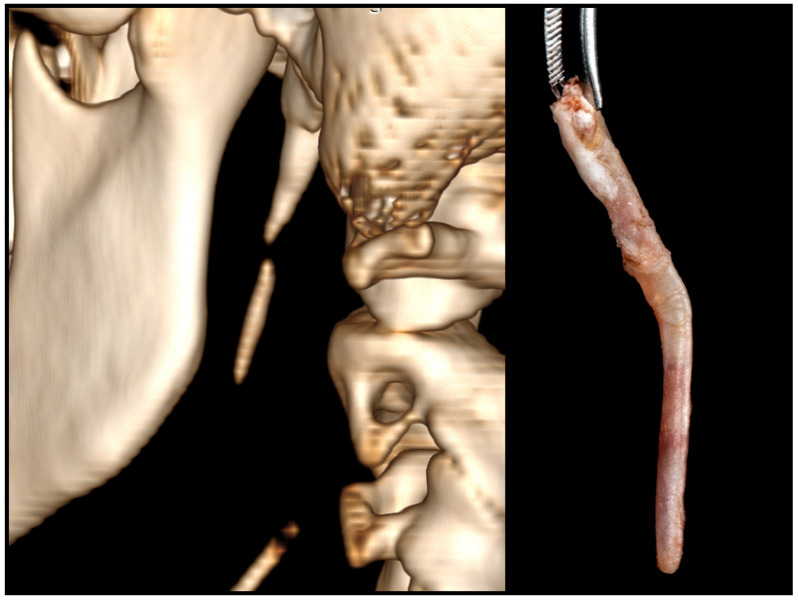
Bony fragments of the stylohyoid chain, individually observed on the 3D CT scan, appeared structurally connected by a calcified ligament upon removal.
